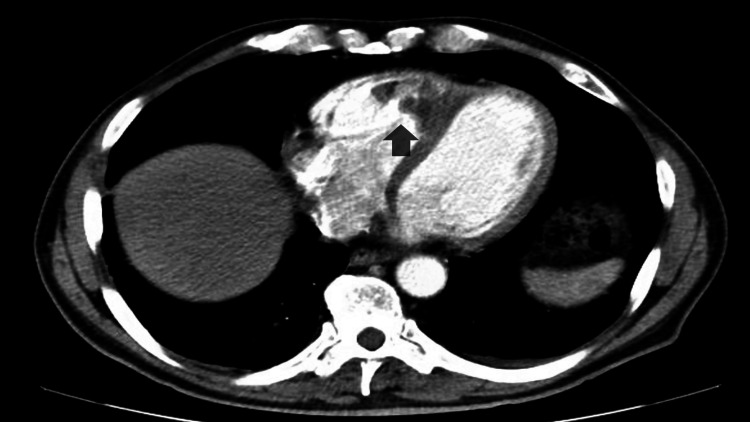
CT scan of the chest with IV contrast showing lobulated mass within the right ventricle (the arrow is pointing toward the intra-cardiac tumor)
